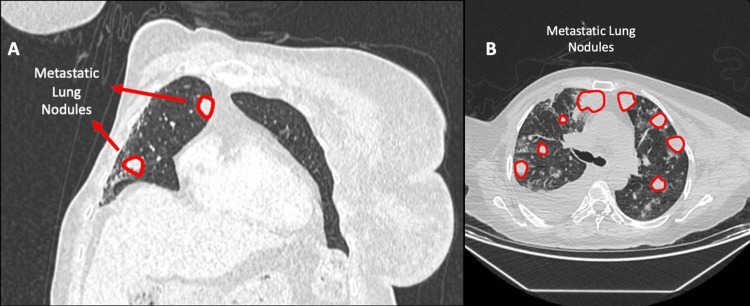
Post-contrast CT images demonstrating the progression of pulmonary metastases A) Post-contrast coronal CT image showing metastatic nodules in the right lung B) Post-contrast axial CT image obtained three months later demonstrating multiple metastatic nodules in both lungs
